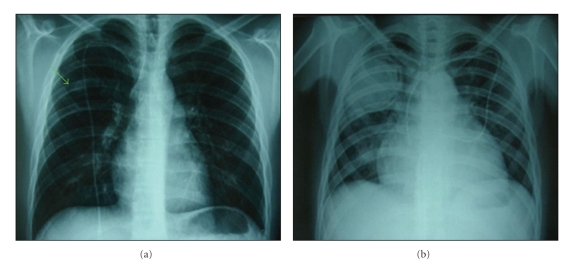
Thoracic X-ray: (a) on day 176, it could be observed a small pulmonary node behind the fifth right thoracic rib (arrow); (b) 3 months, later the pulmonary node was much larger and became cavitated.
